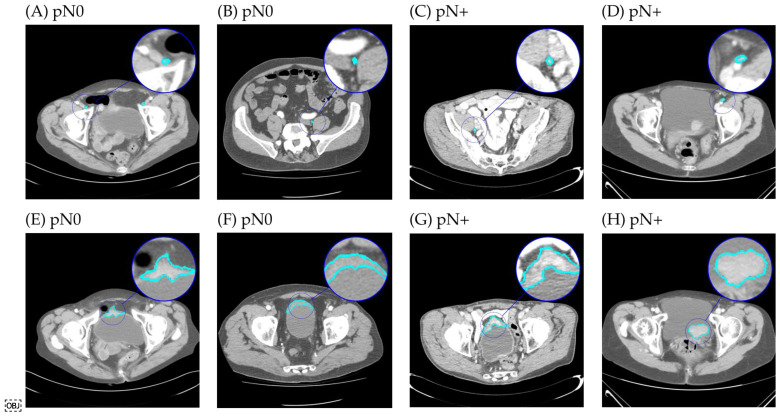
Randomly selected example segmentations on computed tomography scans of four patients with muscle-invasive bladder cancer scheduled for radical cystectomy and pelvic lymph node dissection. Top row: segmentations of pelvic lymph nodes in four patients without (pN0) (A,B) and with (pN+) (C,D) nodal metastases at pelvic dissection. Bottom row: corresponding segmentations of the largest 2D axial cross-sectional area of the primary tumor (E–H).
